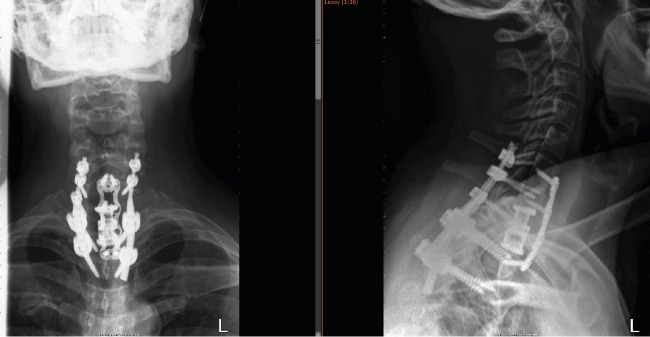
Postoperative AP and lateral X-ray after the second stage of surgery.
